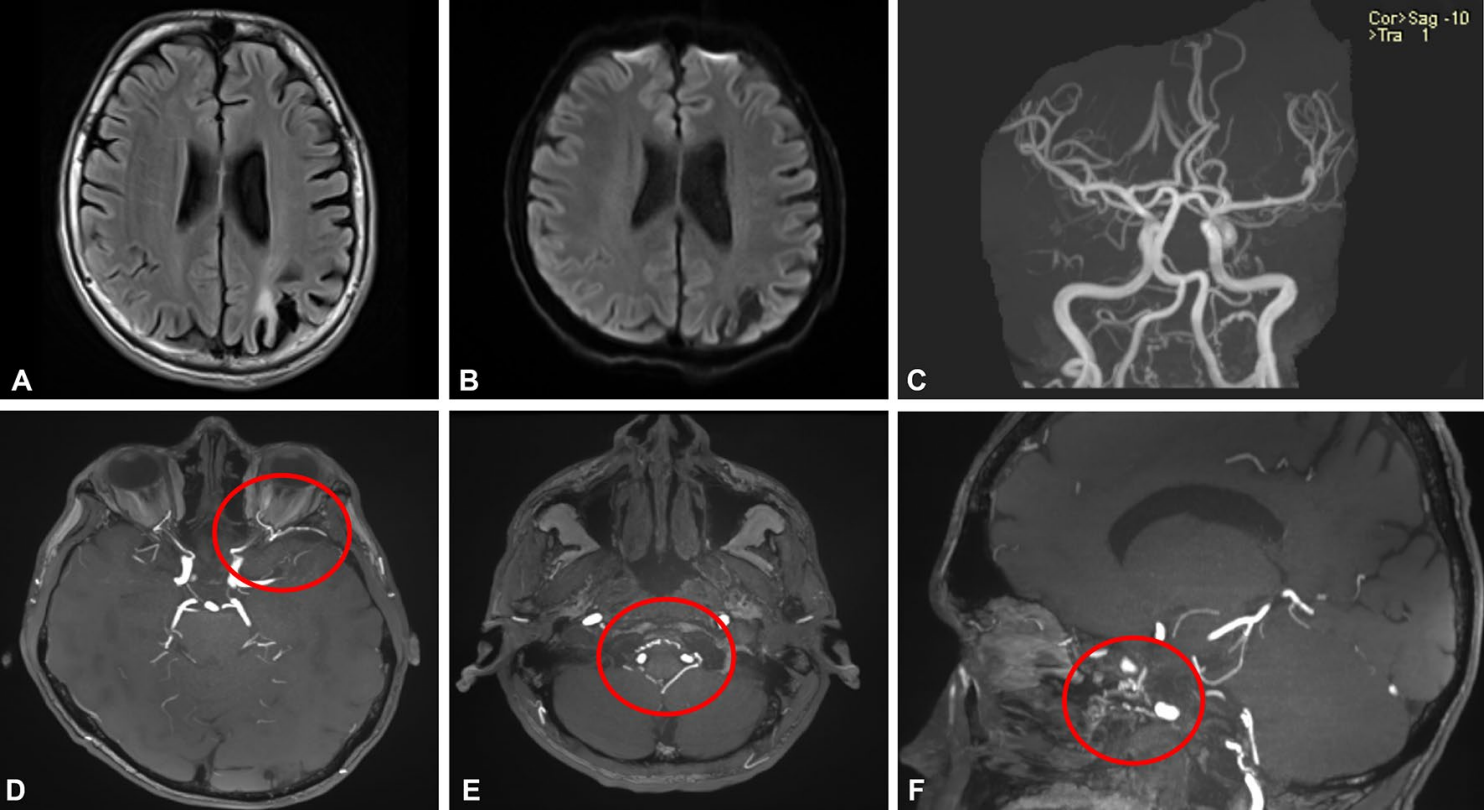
Brain MRI/MRA of a patient with recurrent cerebrovascular events. Axial brain MRI with fluid-attenuated inversion recovery (FLAIR) sequence showing an hyperintense lesion in the distribution of the left middle cerebral artery (a) without demonstrating restricted diffusion in the diffusion-weighted imaging sequences (b), compatible with the patient’s history of prior ischemic strokes. No new lesions were identified on the diffusion weighted imaging sequence of brain MRI confirming the diagnosis of a transient ischemic attack. Large intracranial vessels appeared normal on brain MRA (c). Anastomosis between the left ophthalmic artery and left middle meningeal artery was identified on thin Maximum Intensity Projection (MIP) MRA (circle; d). Meningeal branches in the area of craniocervical junction were dilated (circle; e). Significant dilatation of the branches of the internal carotid artery in the pterygoid canal was also visualized (circle; f)
